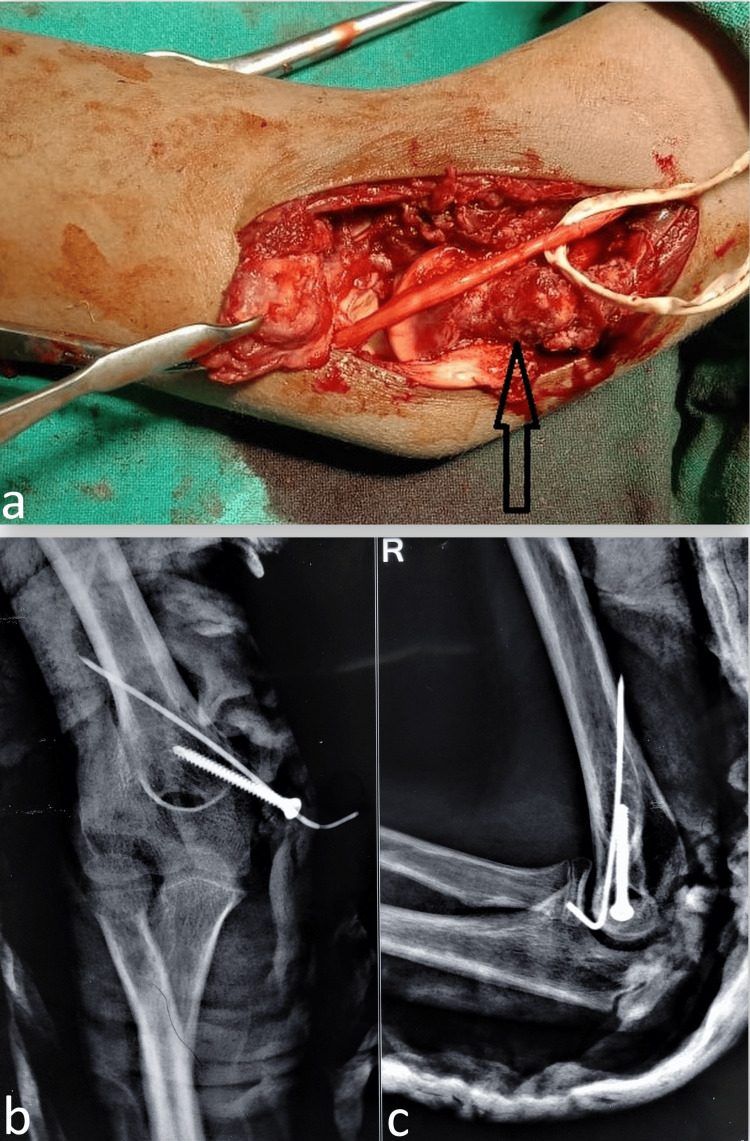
The intraoperative image shows the displaced medial epicondyle fragment (denoted by the tip of the bone lever) lying anteriorly (a) and exploration of the ulnar nerve done before the anterior transposition. The native bed (black arrow) was cleaned before the fixation of the medial epicondyle back to its normal location. The postoperative orthogonal radiograph shows the fixation of the fragment with a K-wire and a screw (b, c).
